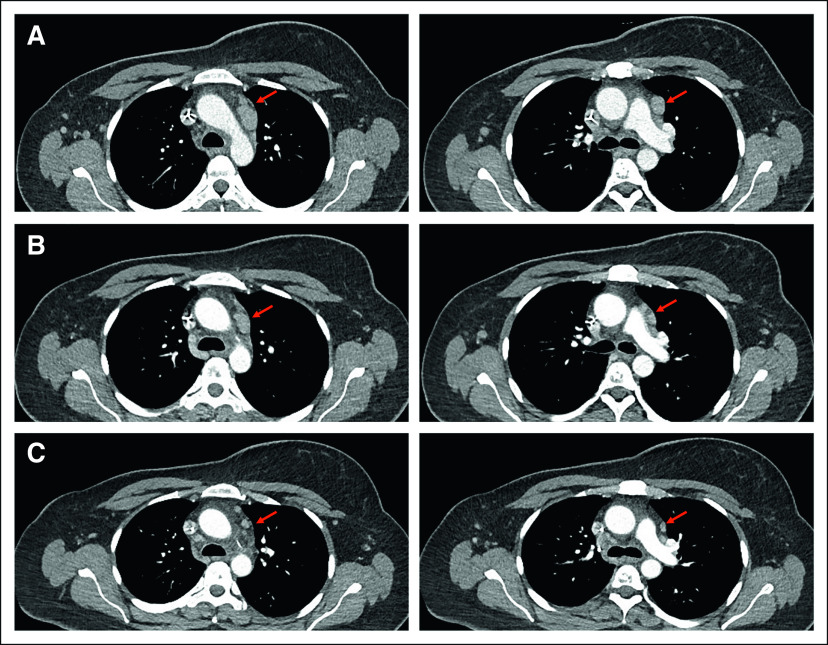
Efficacy of MP0274 in an HER2-positive *PI3K* wild-type breast cancer patient. The patient experienced a sustained partial response in lymph node lesions. Computed tomography scans obtained at (A) baseline and (B) during treatment with 3-week cycles of MP0274 (12 mg/kg) show shrinkage of the lesion at the first scan 2 months later. (C) This tumor reduction was maintained after 14 months of treatment. HER2, human epidermal growth factor receptor 2.
